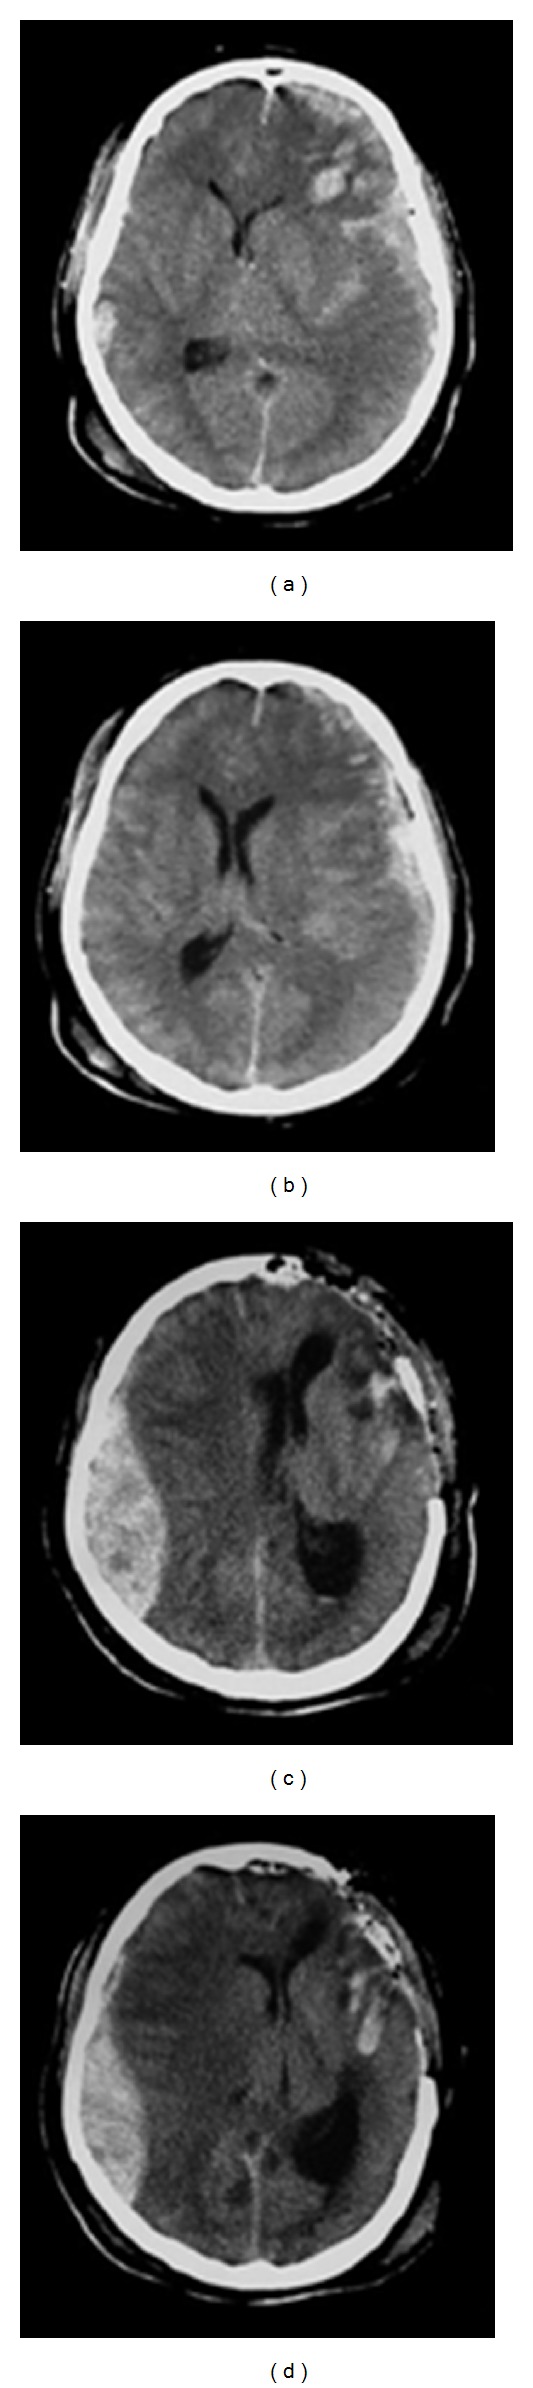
Case 2. Progressive epidural hematoma in a 51-year-old man after motor vehicle collisions. (a) and (b): Initial CT scans showing a left acute subdural hematoma with ventricular compression and midline shift to the right. (c) and (d): Repeat CT scans 16 hours aftertrauma and 13 hours after left frontal-temporal craniectomy; a contralateral epidural hematoma is revealed.
